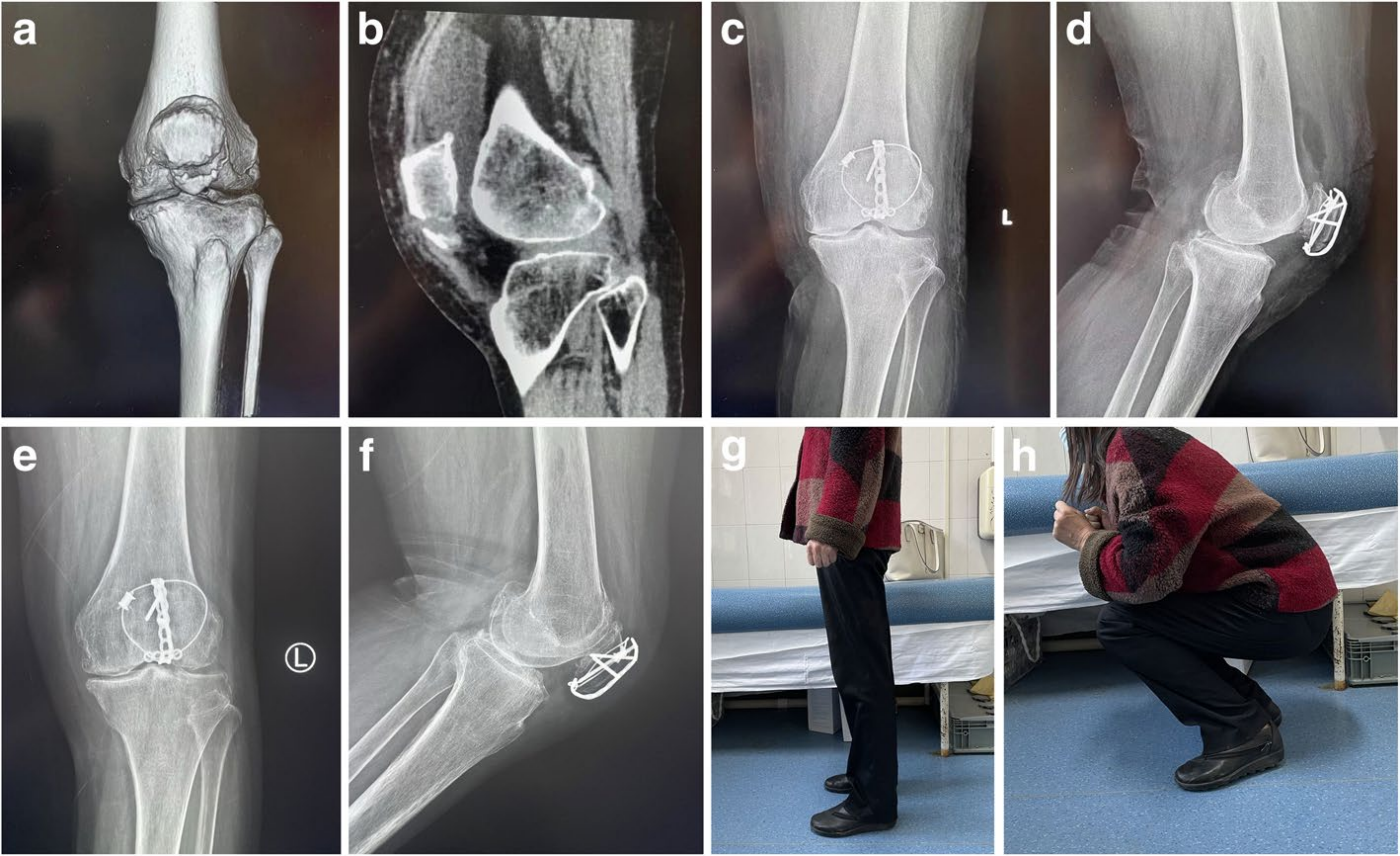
A 53-year-old male suffered a fracture of the inferior pole of the left patella due to a fall. a-b Preoperative three-dimensional CT; c-d anteroposterior and lateral X-ray films of the knee joint of the affected limb on the second day after the operation; e-f One year after the operation, the patient’s anterior and lateral X-ray films of the knee joint; g-h functional appearance of the knee joint 1 year after the operation
